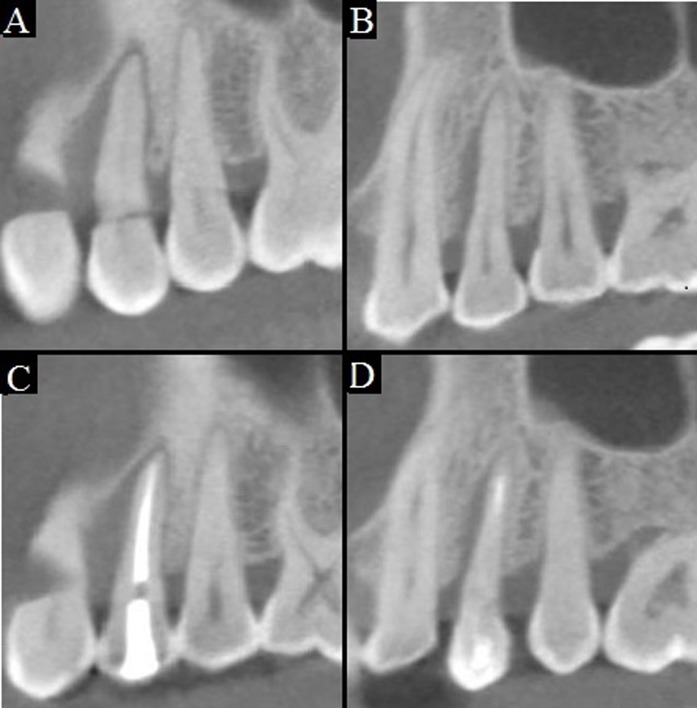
A-B: CBCT images of the vestibular and palatal root previous to the surgical treatment. C-D: CBCT images of the vestibular and palatal root 6 month after the surgical treatment, showing no bone gain.
